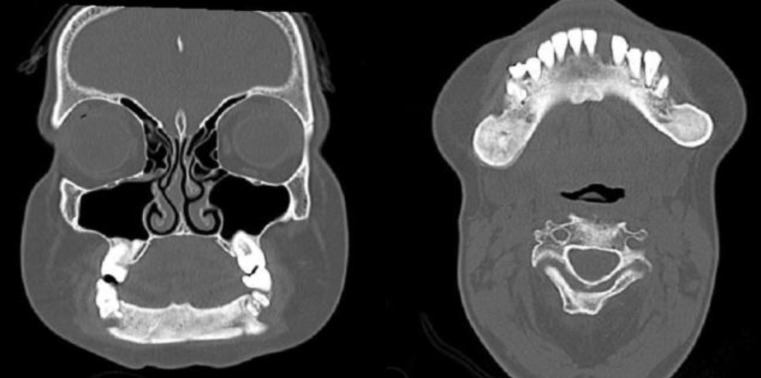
Face CT scan with bone windowing in coronal and axial sections showing homogeneous diffuse bone sclerosis of the mandible, with fat obliteration of bone marrow without cortical thickening
